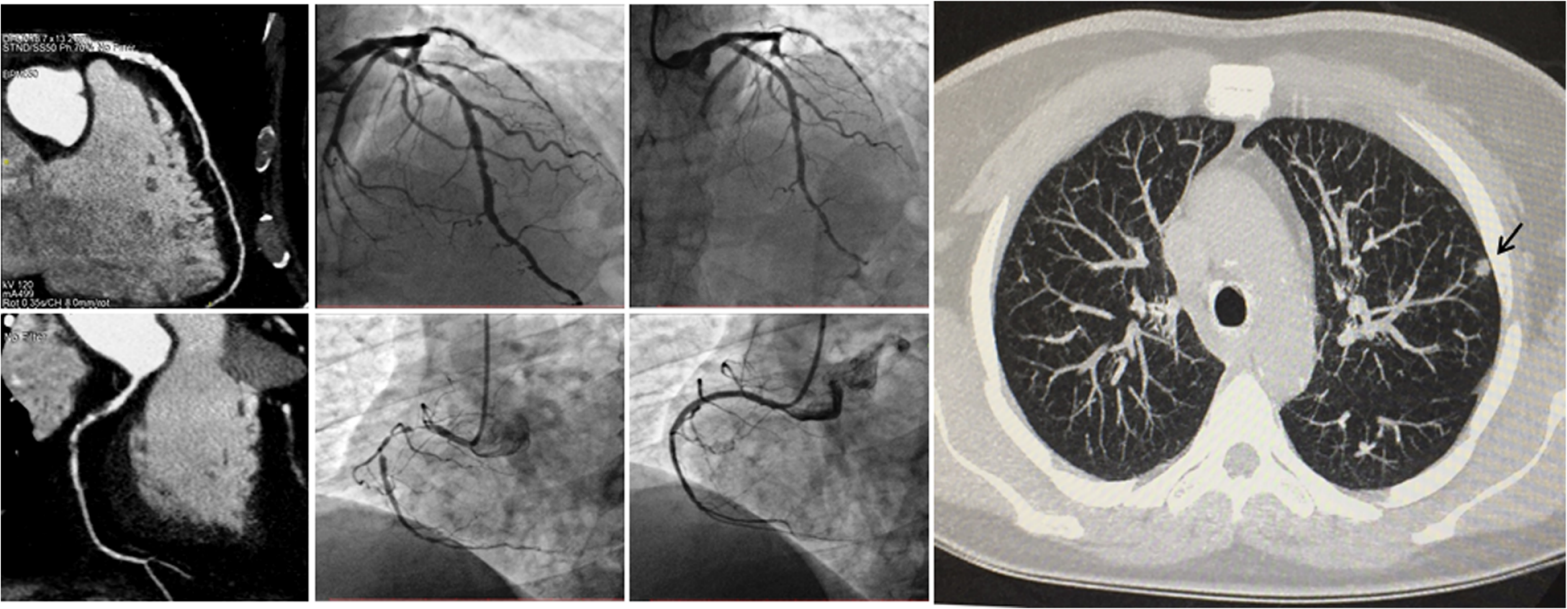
Representative case of coronary artery disease and lung cancer screening. The cardiac CT revealed a significant stenosis of the left anterior descending coronary artery (left upper panel), which was confirmed at coronary angiography (middle upper panel) and treated with percutaneous coronary intervention and stenting (right upper panel). Also, the right coronary artery showed a significant long stenosis in the proximal segment (left lower panel) that was confirmed at coronary angiography (middle lower panel) and treated with percutaneous coronary intervention and stenting (right lower panel). Ultra-low-dose CT images of the lungs showed a 6 mm pulmonary nodule in the left upper lobe (arrow, right panel)
